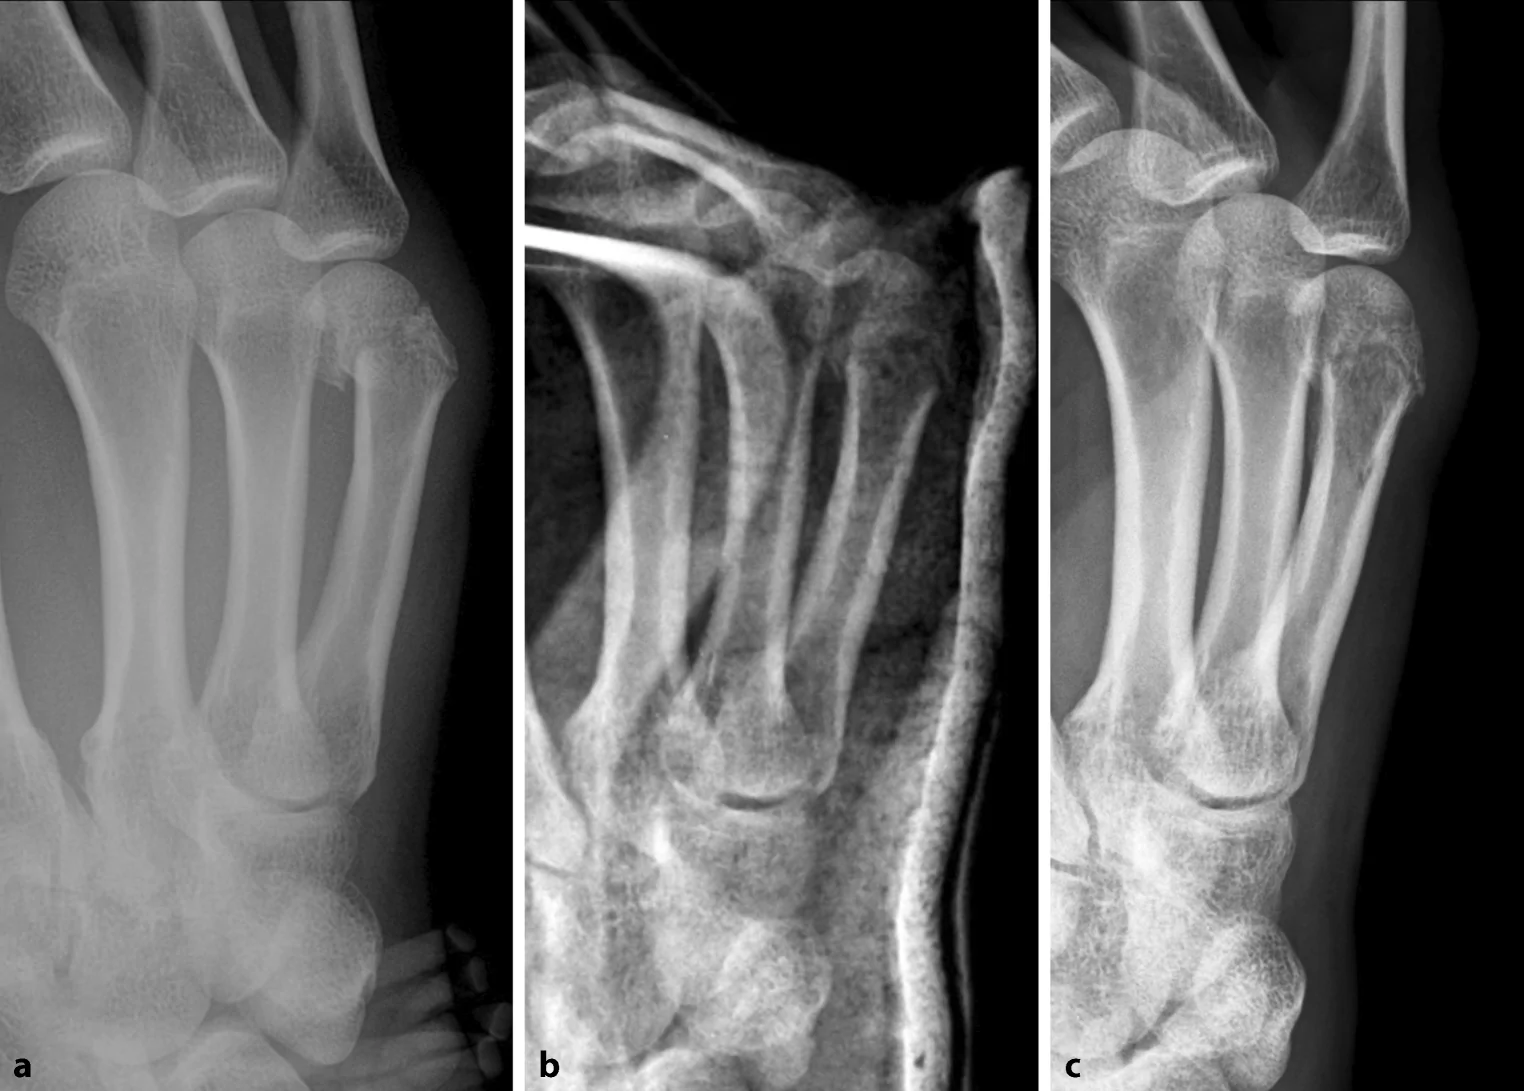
Radiographs of the fingers of a single patient during course of treatment—at initial presentation after trauma (a), with noticeable reduction in the cast (b) and consolidated in improved fracture angulation (c)
